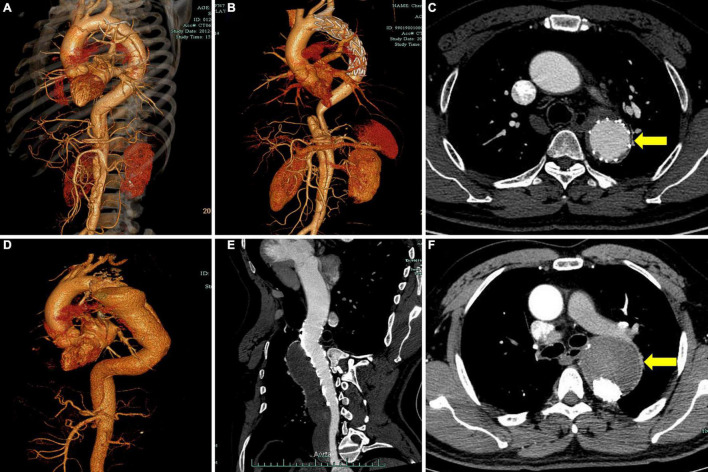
Representative cases of different degrees of FL thrombosis after TEVAR. Computed tomography angiography (CTA) images show the FL status of a patient with subacute aortic dissection before operation (A) and 2 years after TEVAR (B). The cross-sectional image shows complete obliteration of FL (C, yellow arrow). CTA images show the FL status of a patient with chronic aortic dissection before operation (D) and 2 years after TEVAR (E). The cross-sectional image shows total FL thrombosis (F, yellow arrow). FL, false lumen; TEVAR, thoracic endovascular aortic repair.
